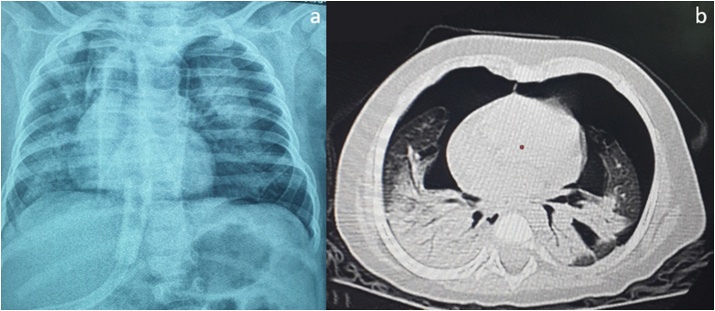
a. CXR showing left pneumothorax with foci of condensation. b. Axial sequence of a lung window chest-CT revealing a bilateral large pneumothorax with an interstitial alveolar pneumonitis and posterior consolidation.
